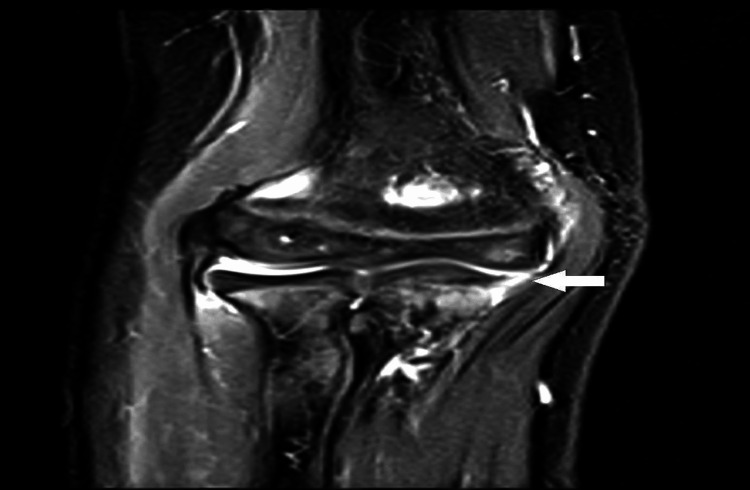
MRI of the elbow showing partial tear of the medial ulnar collateral ligament (arrow). MRI, magnetic resonance imaging
